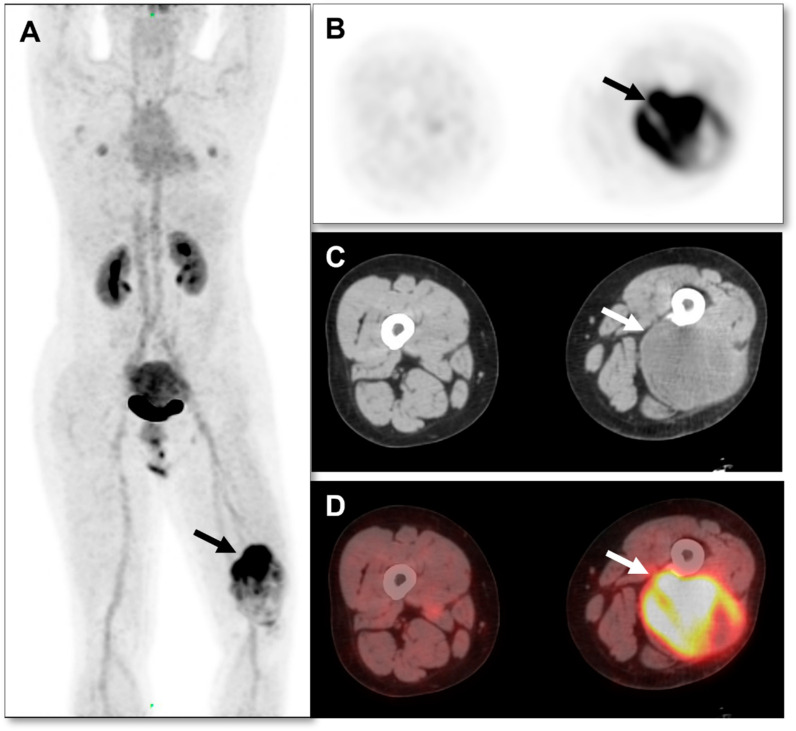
^68^Ga-FAPI PET/CT was performed 2 days after ^18^F-FDG PET/CT. ^68^Ga-FAPI PET/CT MIP image (A), arrow indication showing abnormal ^68^Ga-FAPI high radiotracer uptake mass in the posterior muscle of the left lower thigh, SUVmax: 11.3. MIP image does not show lymph nodes or distant metastases. It is evident from the axial images ((B), CT; (C), PET; (D), fusion PET/CT) that FDG uptake is overall weak and more homogeneously distributed than ^68^Ga-FAPI, which conversely has a large focus of high uptake in the cranial half of the mass and a weaker uptake in the remainder. ^68^Ga-FAPI-derived SUVmax was significantly higher than ^18^F-FDG PET/CT (11.3 vs. 6.8, arrow indication). No significant abnormal FAPI radiotracer uptake was observed in other parts of the patient. This patient was considered for sarcoma with FDG and FAPI imaging.
